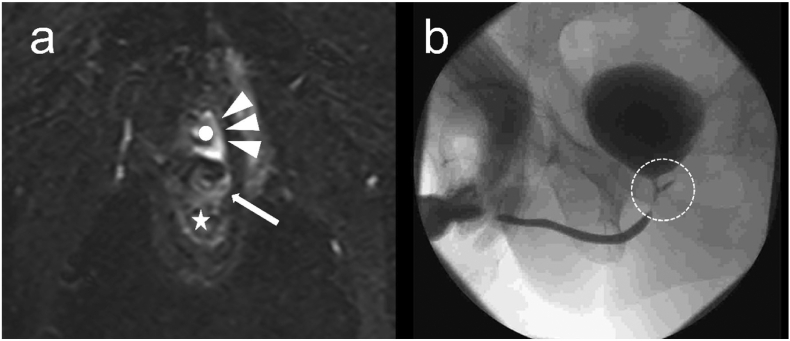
Examination findings at 5 months after cystostomy and ileostomy for RUF. a) MRI: A fistula (arrow) and fluid accumulation around the urethra (arrowhead) were observed between the rectum (☆) and urethra (○). b) Cystography: Contrast leakage was observed on the dorsal side of the urethra (dotted circle).
